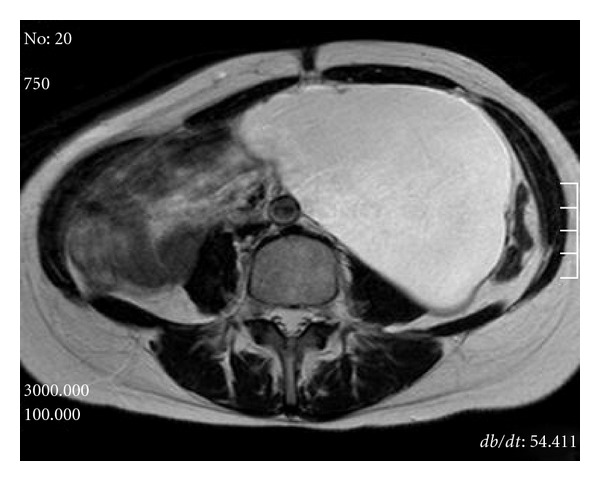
Magnetic resonance imaging showing a large simple cystic retroperitoneal mass.
